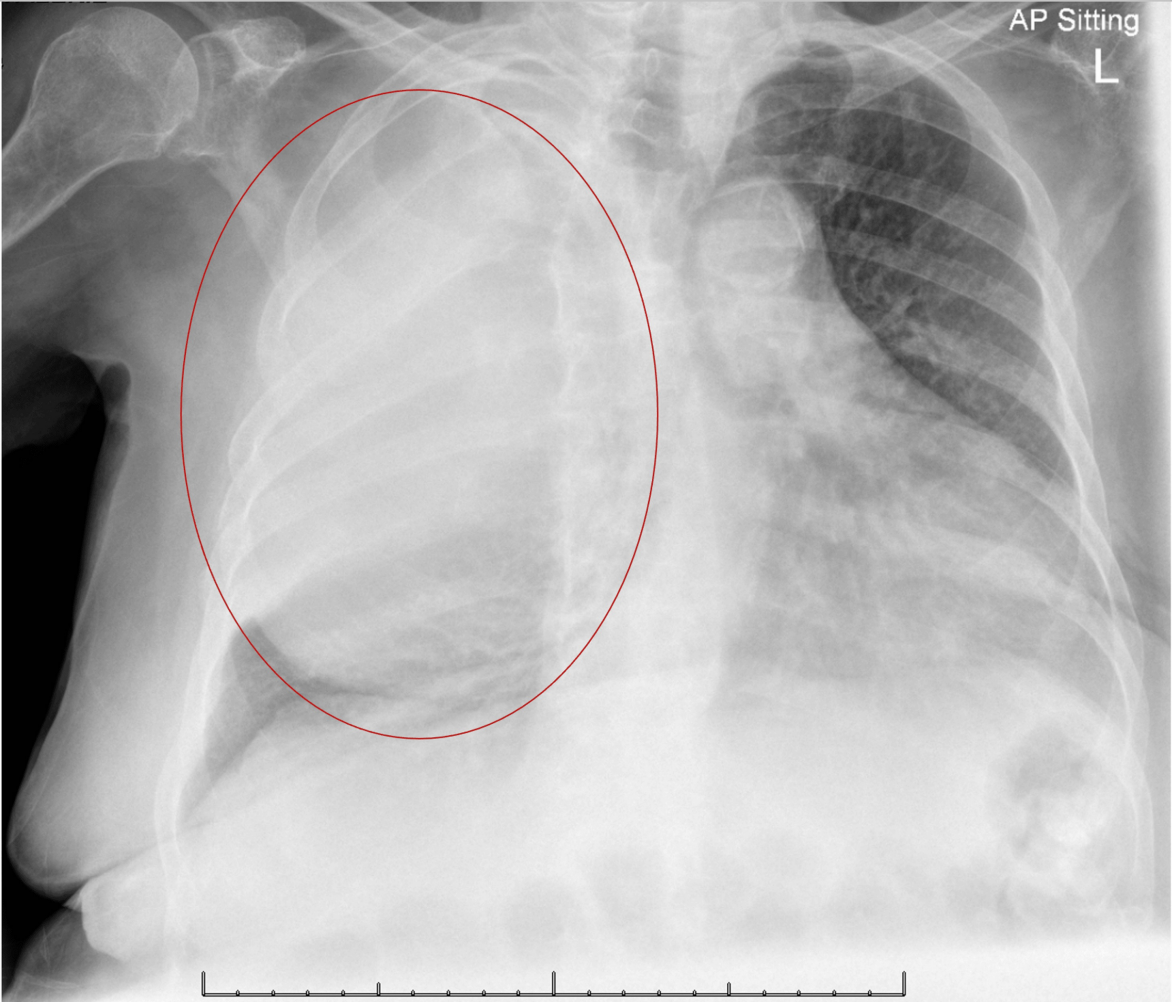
AP sitting chest X-ray AP: Anteroposterior
